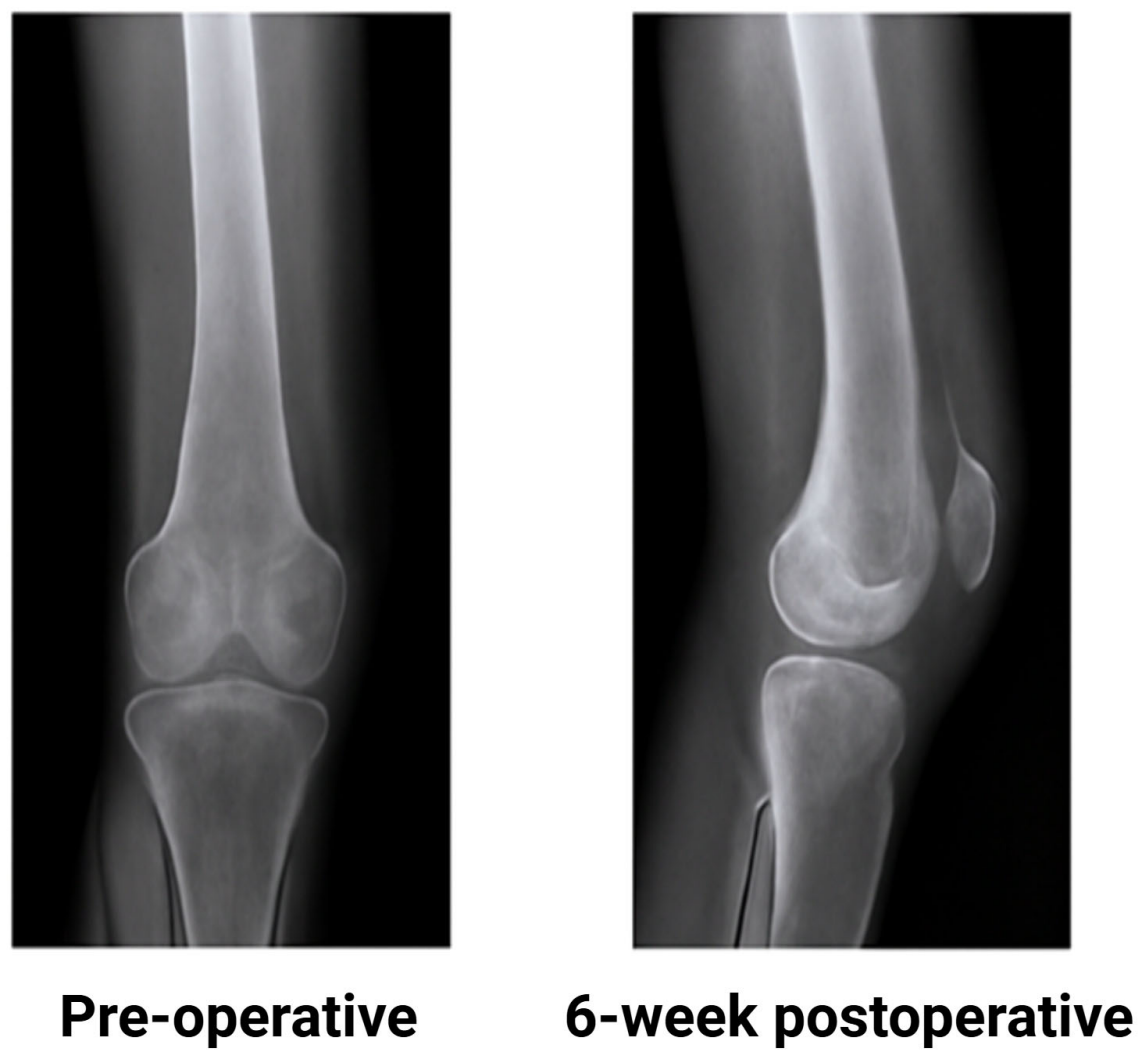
Radiographic analysis of bone healing.
